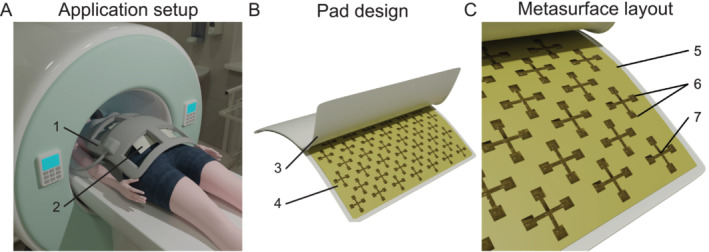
(A) Conceptual image of MRI scanning of the abdominal cavity with passive shimming: The receive body array (1) is placed on a volunteer with the pad (2) on the fetal area. (B) A conceptual image of a case (3) with metasurface (4). (C) Metasurface design (top layer): dielectric substrate (5) with parallel plate capacitors (6) connected by metal crosses (7).
